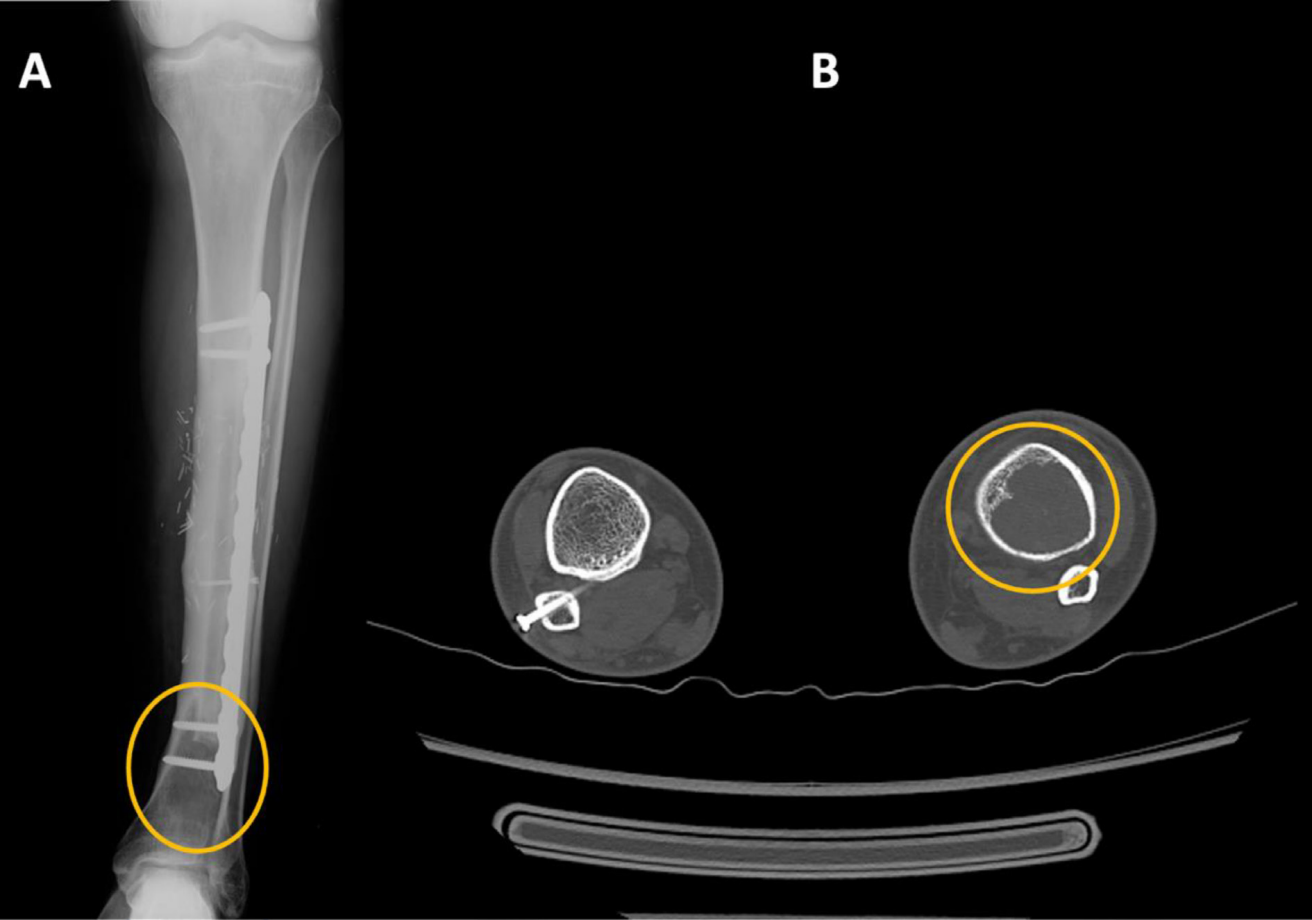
X-ray (A) and CT evidence (B) of an osteolytic lesion localized in the left distal tibia.
